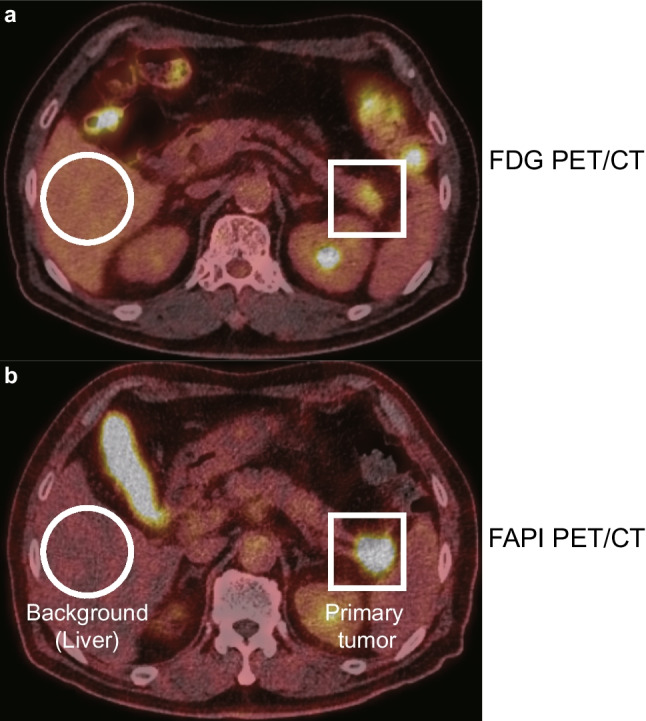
(a) [^18^F]FDG and (b) [^18^F]AIF-FAPI-74 PET/CT images of a 72-year-old man with newly diagnosed pancreatic tail cancer for tumor staging
